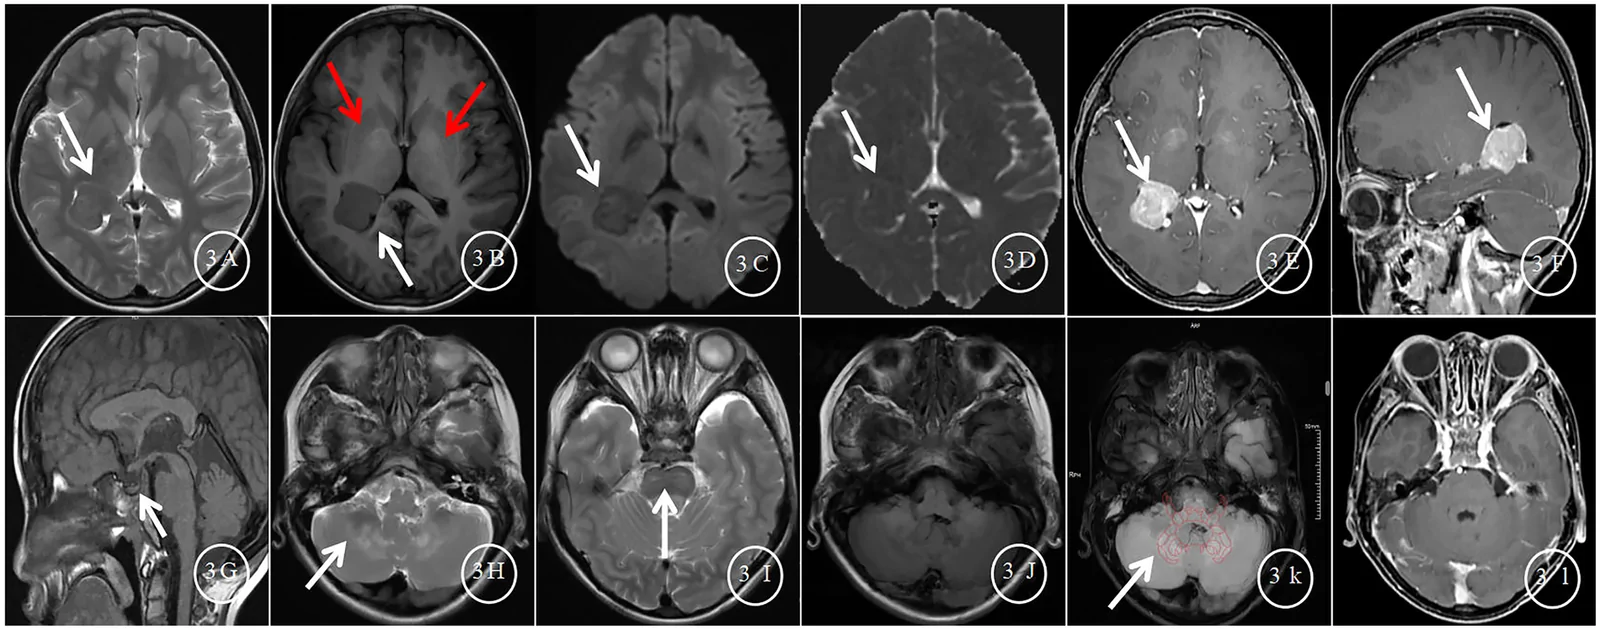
MRI findings of ECD. (A,B) Axial T1-weighted (T1W) and T2-weighted (T2W) images: a round lesion in the right lateral ventricle trigone showing slightly hypointense signal on T1WI and iso-to-slightly hyperintense signal on T2WI (white arrow); patchy hyperintense signals in the bilateral basal ganglia (red arrows). (C,D) Diffusion-weighted imaging (b = 1,000 s/mm^2^): no restricted diffusion (white arrow). (E,F) Contrast-enhanced T1WI: marked homogeneous enhancement of the ventricular lesion. (G) Sagittal T1-weighted non-contrast image: normal sella turcica with absence of the posterior pituitary bright spot (white arrow). (H–K) Axial T1WI and T2WI images: ill-defined lesions in the bilateral cerebellar hemispheres, pons and cerebral peduncles, appearing hypointense on T1W and hyperintense on T2WI/FLAIR, resembling a “crab” (K, white arrow). (L) No enhancement on post-contrast T1WI.
